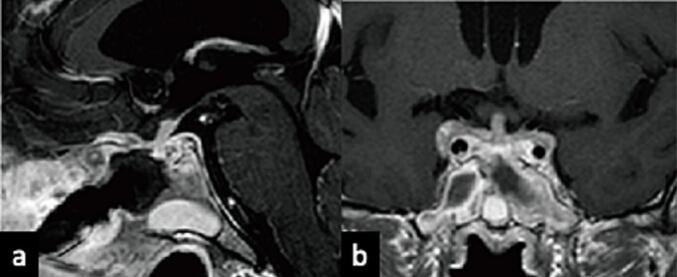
T1-weighted image with contrast after the radiation therapy showing tumor reduction (a: sagittal, b: coronal).
